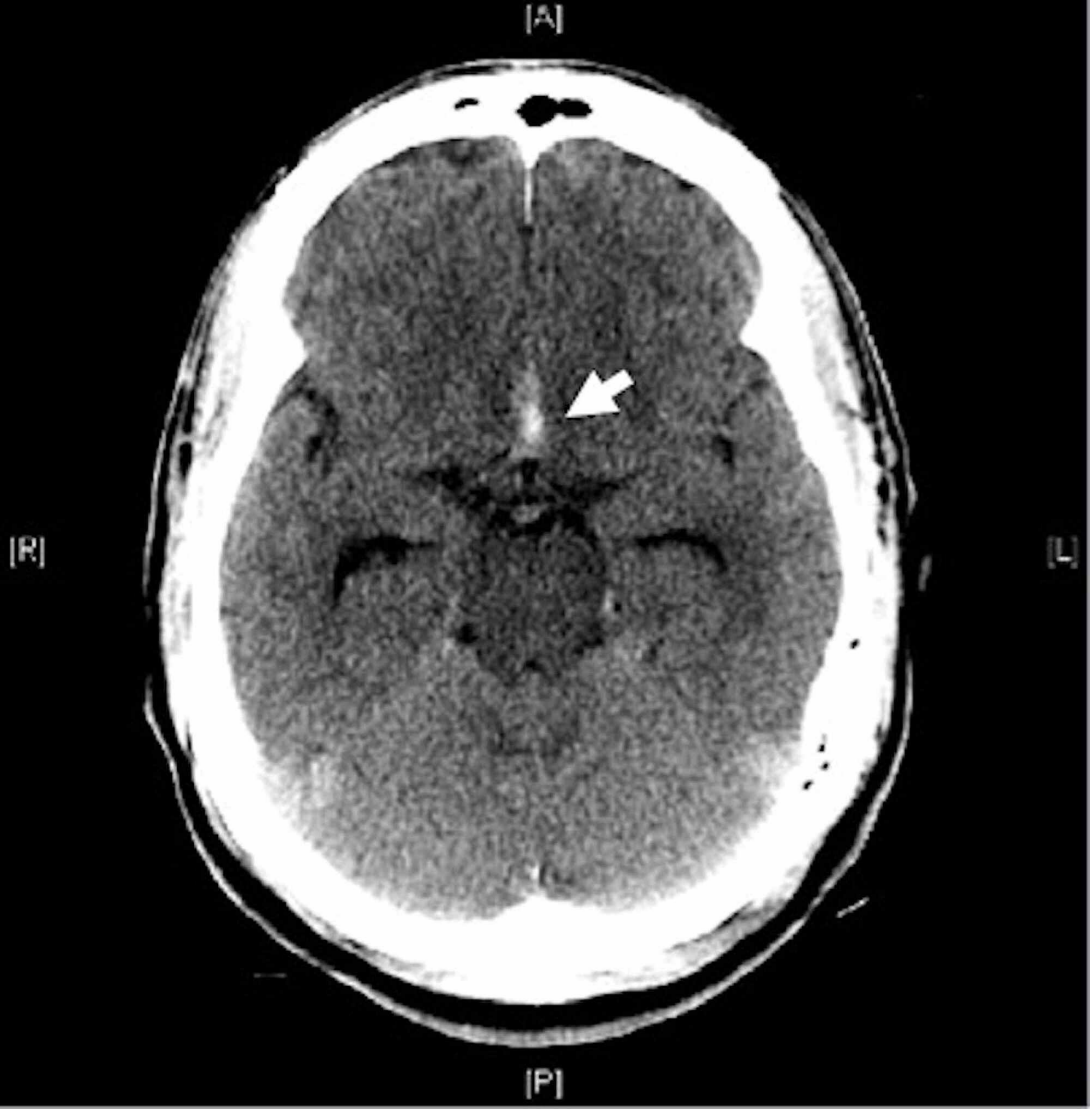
Axial non contrasted CT of the brain showing subarachnoid hemorrhage in interhemispheric fissure (arrow).
